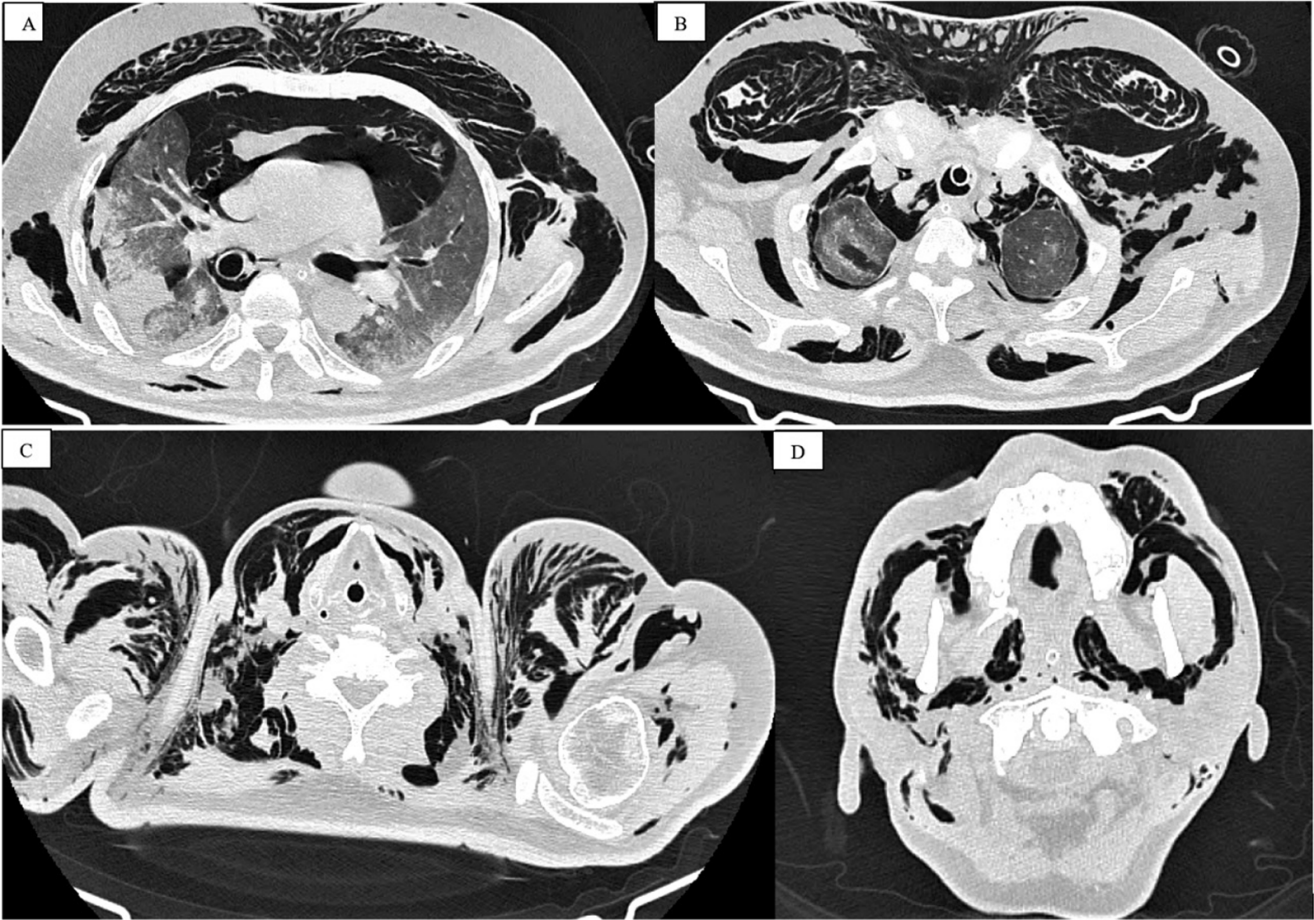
A 56-year-old male was brought to our hospital with worsening shortness of breath. The patient was moved to the critical care unit after failing to maintain a SpO2 of over 87 percent on the fourth day of hospitalisation (ICU). Within 3 days of intubation, axial CT thorax sections indicated severe barotrauma in the form of pneumomediastinum, pneumothorax, and subcutaneous emphysema (A, B and C). Subcutaneous emphysema had also spread to the neck and face (D)
